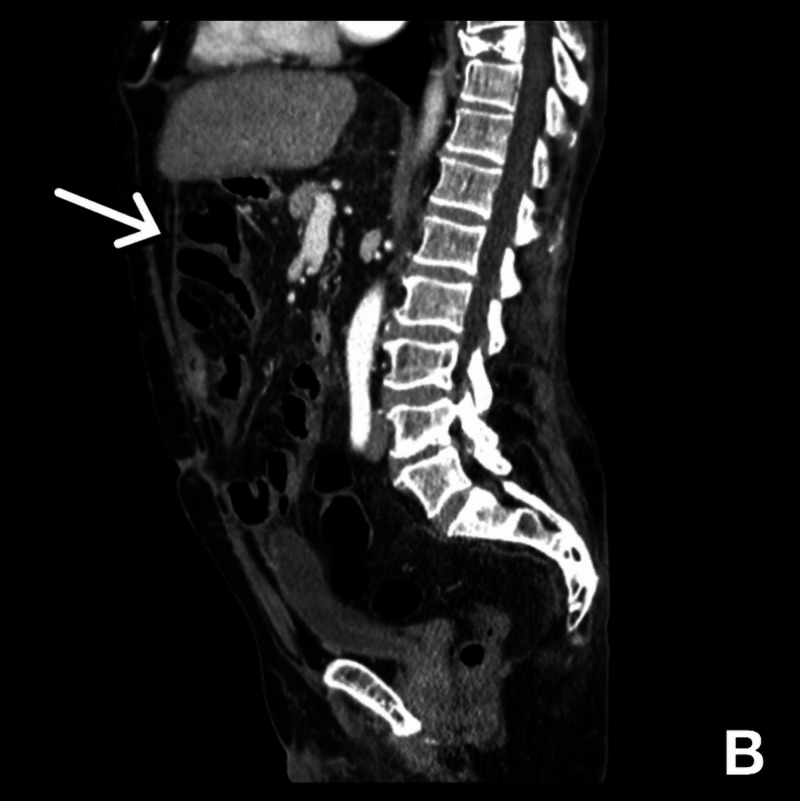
Sagittal view of CT of the abdomen, with arrow pointing to fibrous tissue encasing the stomach, small intestine, gall bladder, omentum, and large intestine
